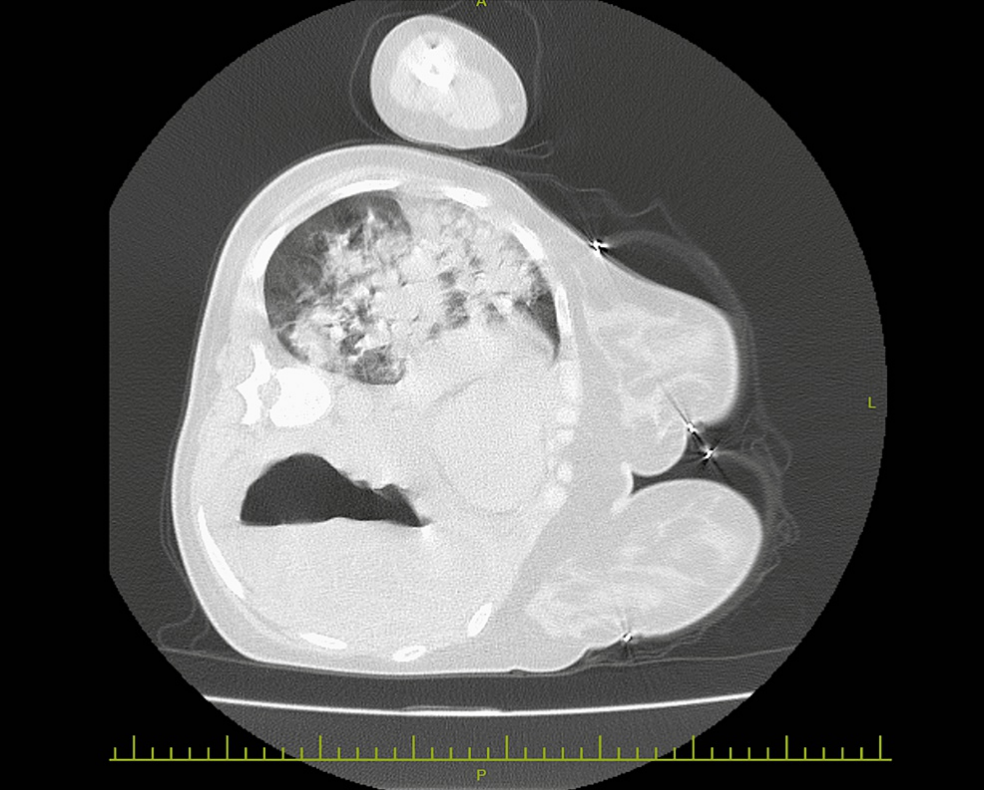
CT scan of the chest (lung window) A giant cystic lesion with an air-fluid level on the left and air bronchogram containing pulmonary consolidation on the right lung.
